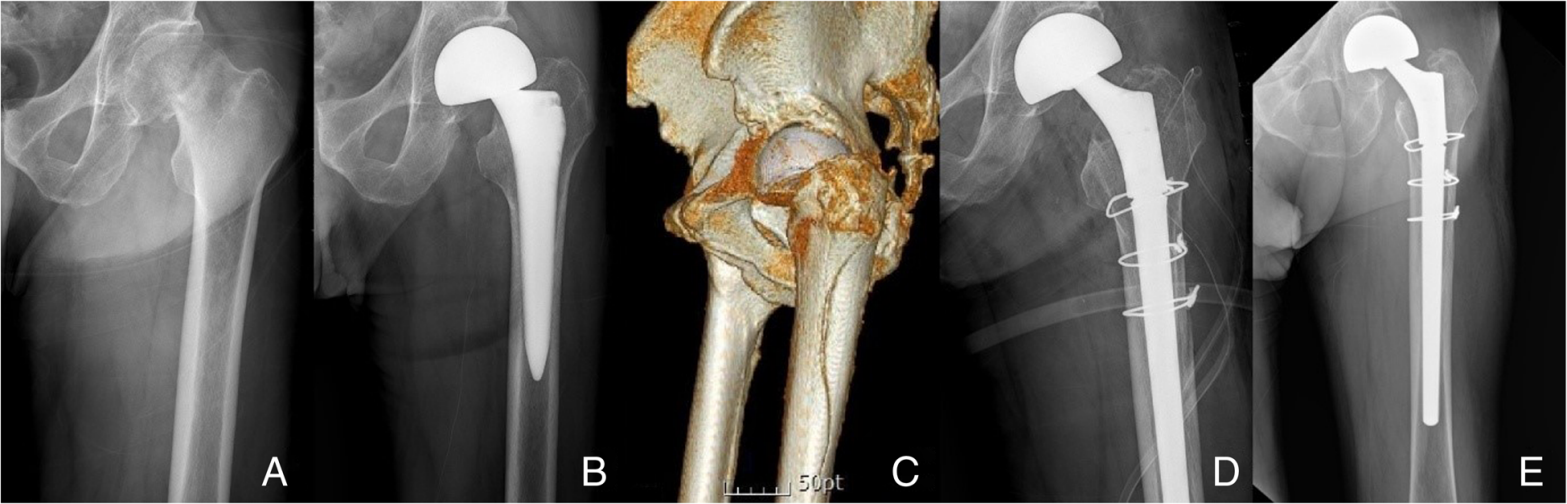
a Preoperative radiograph of a 70-old-year man with subcapital femoral neck fractures. b Postoperative radiograph shows the excellent implant position of cementless bipolar hip arthroplasty. c A Vancouver type B fracture with stem loosening occurred after a fall at 2 months postoperatively. d Stem revision was performed using a long distal-fitting and modular-type stem with additional circular wiring. e At 24 months after revision surgery, the radiograph showed bony union and stable stem fixation
